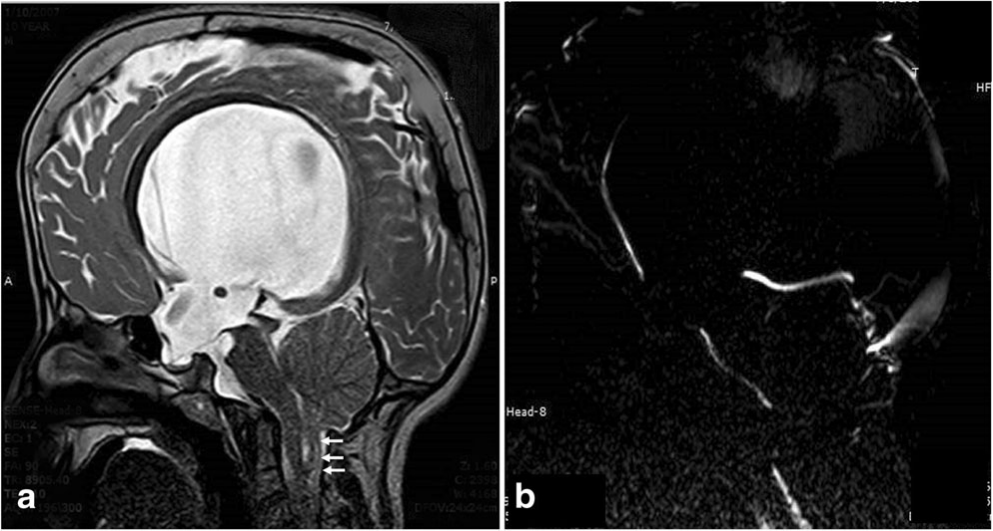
A 10-year-old boy with Chiari malformation. a Sagittal T2-WI showing cerebellar tonsil herniation. b Phase-contrast MRI demonstrates obstructed CSF flow at the foramen magnum
